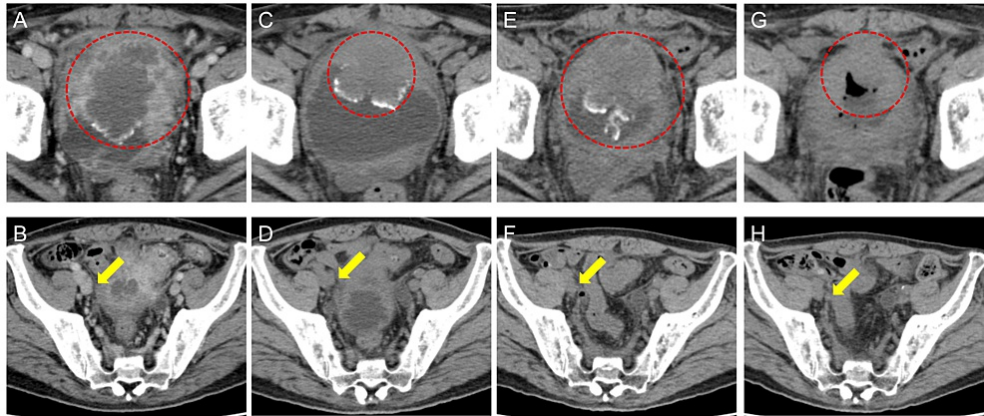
Durable response to enfortumab vedotin and additional radiation therapy in Case 2 Computed tomography (CT) showing shrinkage and increase of the primary bladder tumor (red circle) and lymph node metastasis (yellow arrow) in case 2. (A, B) Pretreatment; (C, D) after two cycles; and (E, F) after three cycles of enfortumab vedotin (EV) therapy. (G, H) After additional radiation with EV therapy, indicating maintenance of tumor shrinkage for the primary ureteral carcinoma and lymph node metastasis was noted.
